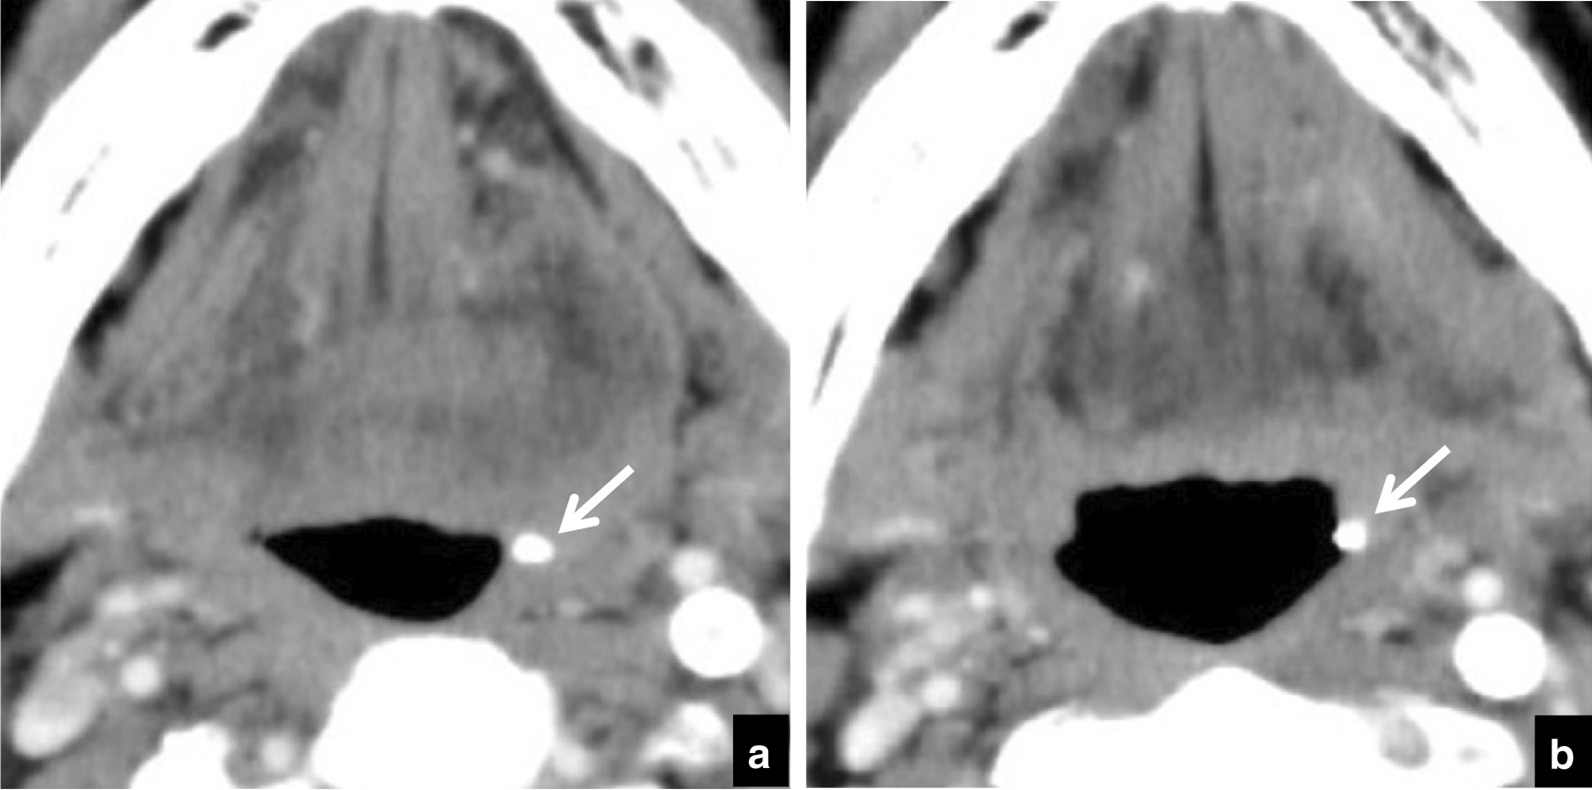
A 60-year-old subject with malignant tumor of the tongue in whom tonsillolith size decreased on CT. a Axial CT images at the palatine tonsillar level at the initial examination. The arrow indicates a tonsillolith. The long axis of the tonsillolith is 5.2 mm. b Follow-up CT image at the same level 18 months later. The long axis of the tonsillolith (arrow) has decreased to 4.3 mm
